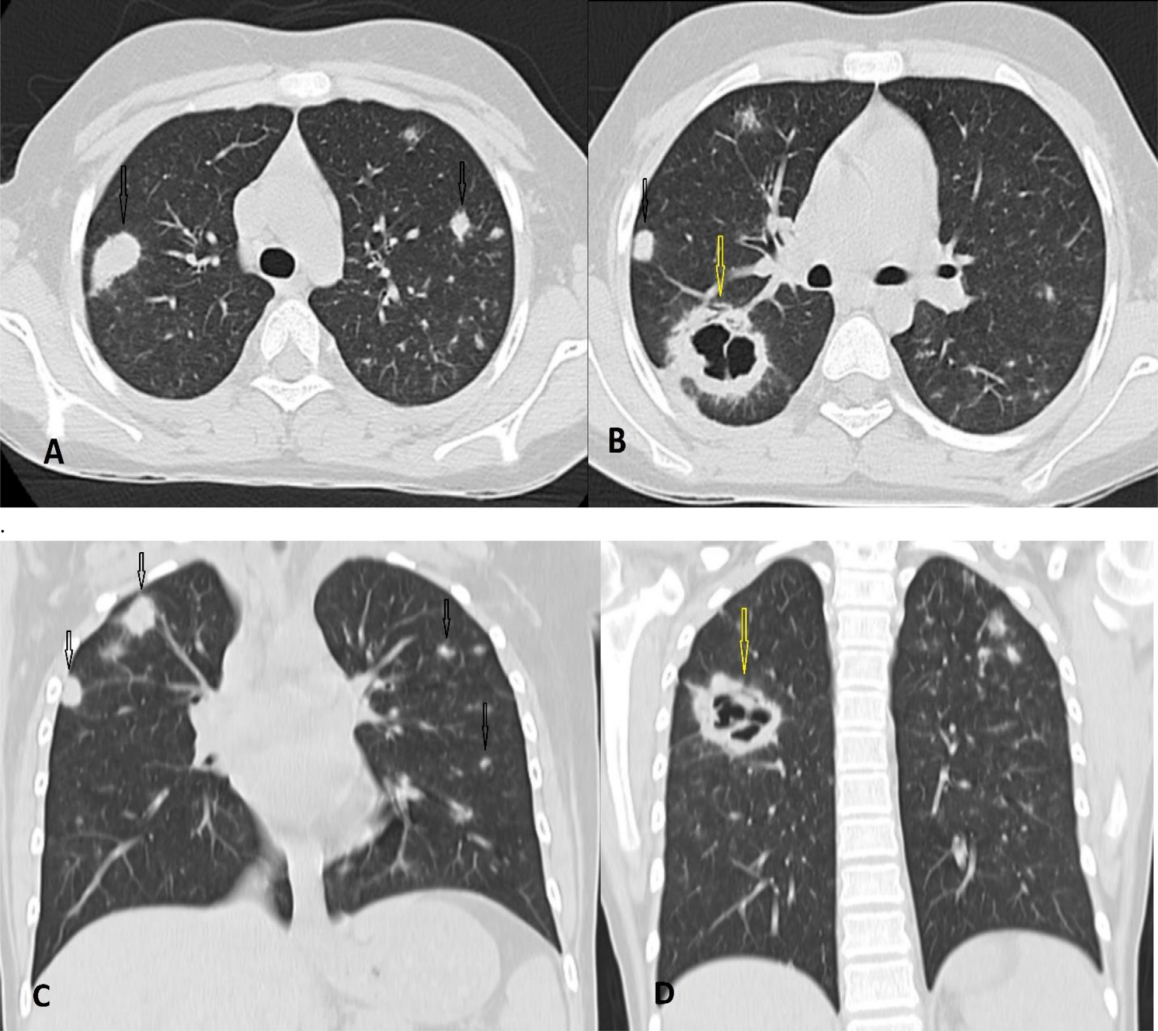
Axial (A, B) and coronal (C, D) lung view of a thoracic CT scan without contrast: Multiple pulmonary nodules and masses in both lungs, affecting the upper and lower lobes, are present. (Black arrows) Some of these masses have central cavitation (yellow arrows). Additionally, some nodules have peripheral ground glass attenuated noted.
